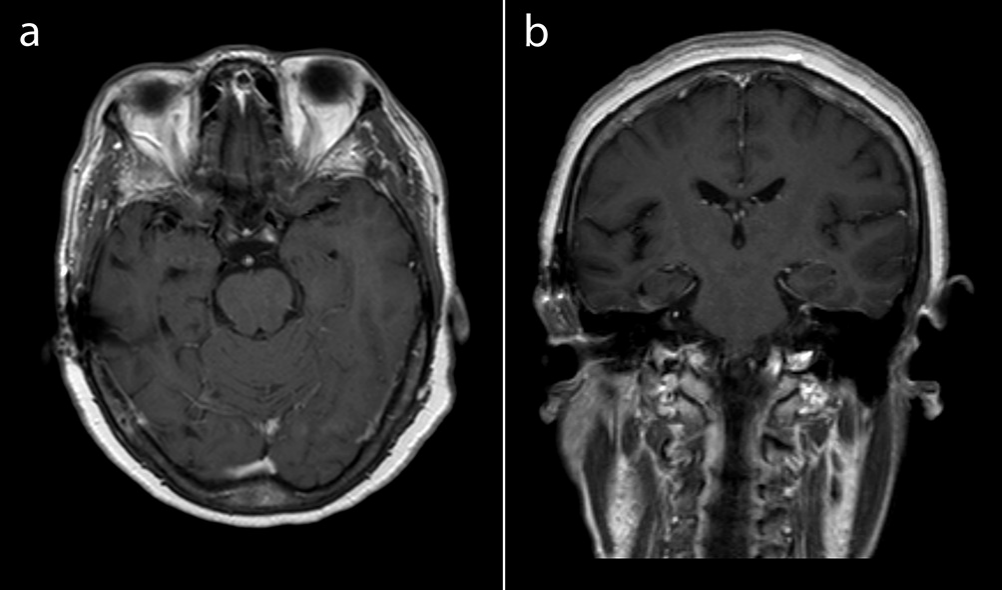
A follow-up MRI performed 2 years later with (a) transaxial and (b) coronal *T*_1_ weighted post-gadolinium images showed complete resolution of the right temporal lobe *Histoplasma capsulatum* lesions.
